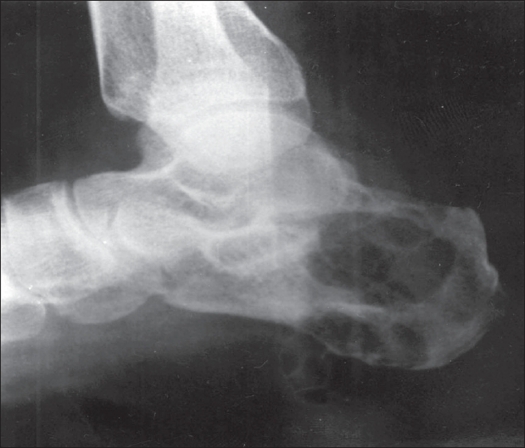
X-ray (lateral view) of the foot showing osteolytic lesion in the calcaneum, with intact articular surfaces
